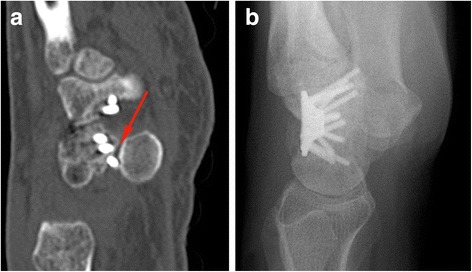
Only the CT scan (a) revealed a screw displacement into the pisotriquetral joint (see arrow), which was not obvious on conventional X-Ray [b, sagittal view]
